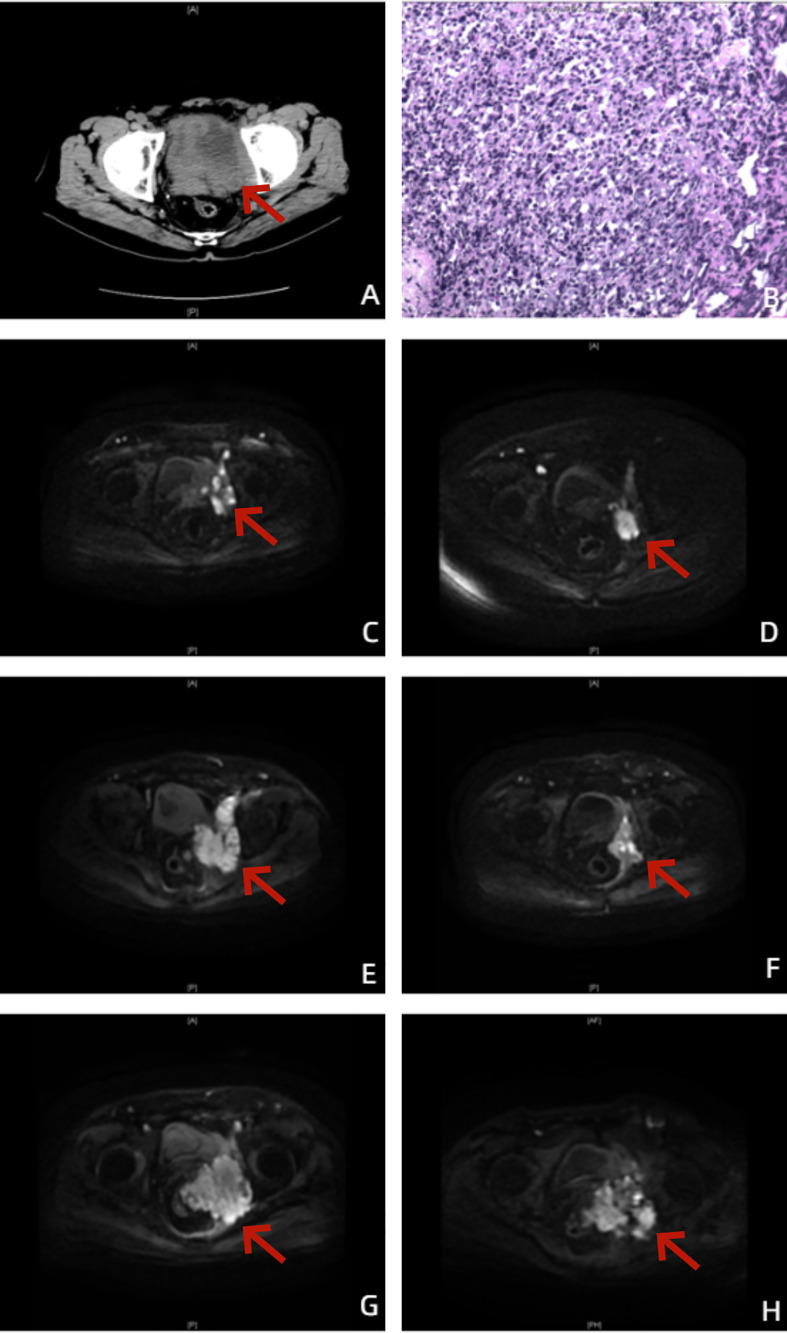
Images of the patient throughout the treatment. (A) Before treatment, urinary tract CT showed a localized mass with mixed density in the pelvic region, unclearly displayed prostate and bladder, enlarged pelvic and retroperitoneal lymph nodes, and mildly dilated bilateral renal pelvis and ureter. (B) The postoperative pathological diagnosis showed prostate adenocarcinoma, Gleason score (GS) 5 + 4 = 9. (C) On 18 June 2020, prostate-enhanced MRI cross-sectional DWI showed an enlarged prostate with a maximum cross-sectional size of 50 × 37 mm, prostate cancer with invasion of the left pelvic sidewall and left side of the bladder, unclearly displayed bilateral seminal vesicles, and enlarged left external and internal iliac lymph nodes. (D) On 15 March 2021, prostate-enhanced MRI cross-sectional DWI showed an enlarged prostate with a maximum cross-sectional size of 50 × 37 mm and a significantly reduced volume of the prostate and left pelvic sidewall lesions compared to before treatment. (E) On 24 May 2021, prostate-enhanced MRI cross-sectional DWI showed a significantly increased lesion volume in the left pelvic sidewall and new metastasis in the left femoral neck, sacrum, and bilateral iliac crest. (F) On 17 November 2021, prostate-enhanced MRI cross-sectional DWI showed prostate with a maximum cross-sectional size of 37 × 29 mm and significantly reduced volume of lesion of prostate and left pelvic sidewall. Except for the left femoral neck, no bone metastases were found in the other parts of the body. (G) On 23 March 2022, prostate-enhanced MRI cross-sectional DWI showed that the volume of the lesion of the left pelvic sidewall was significantly increased, and rectal invasion was not ruled out. (H) On 8 September 2022, prostate-enhanced MRI cross-sectional DWI did not show new confirmed progression of imaging.
